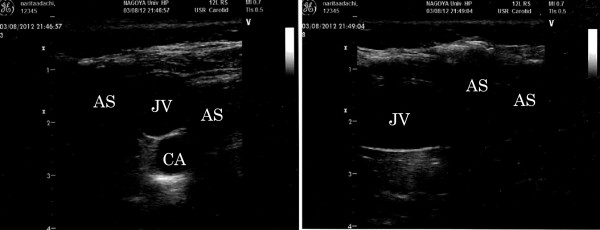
Ultrasound images. Left: The ultrasound image of the patient's neck in coronal view was demonstrated. The identification of both jugular vein and carotid artery was barely possible in spite of many subcutaneous ultrasound barriers by emphysema. Right: The ultrasound image in sagittal view was demonstrated. The jugular vein was feasibly observed; however, the carotid artery that is a relatively deep structure could not be identified. *JV* jugular vein, *CA* carotid artery, *AS* acoustic shadow by air.
